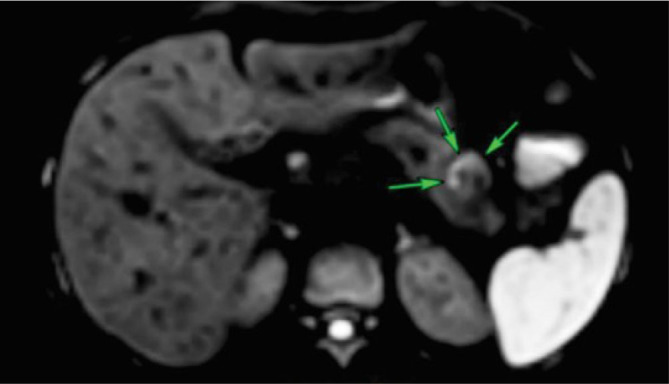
Magnetic resonance imaging six months postoperatively.
